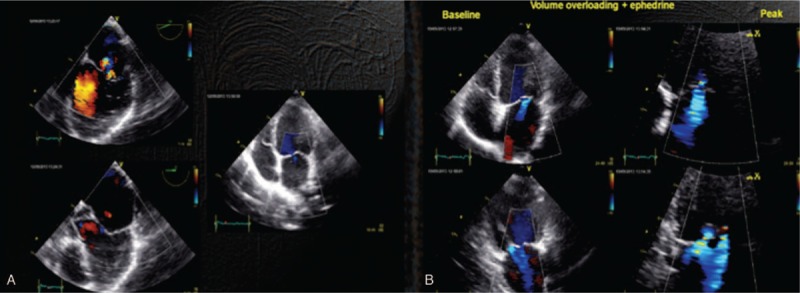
Echo lab. (A) TEE and TTE demonstrate minimal regurgitation. (B) After handling the load (saline and ephedrine) increase in the regurgitation with very obvious area of flow convergence. TEE = transesophageous echocardiogram, TTE = transthoracic echocardiogram.
